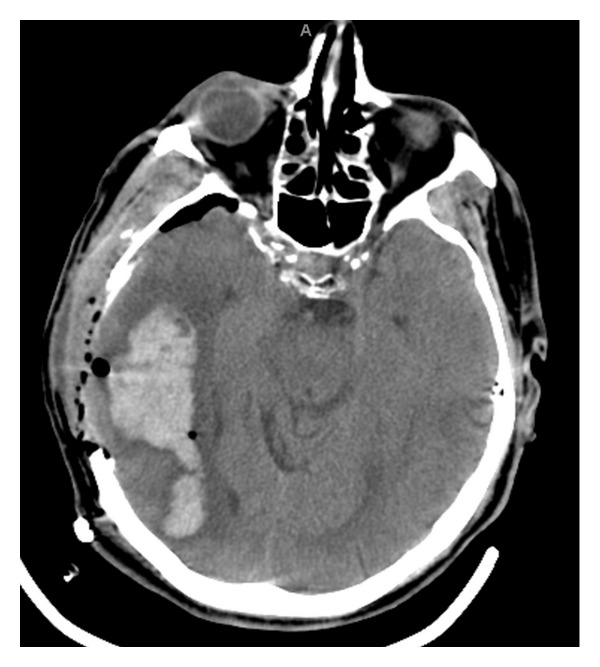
CT of the head immediately after a right sided craniotomy was performed.
